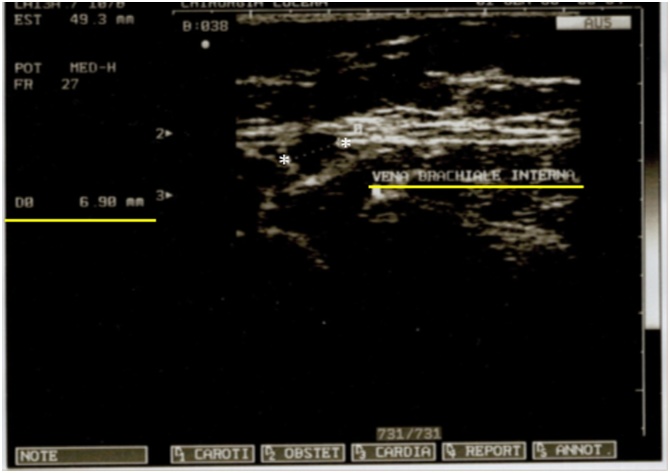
Ultrasound of arm veins; diameter of the brachial vein of the left arm.
